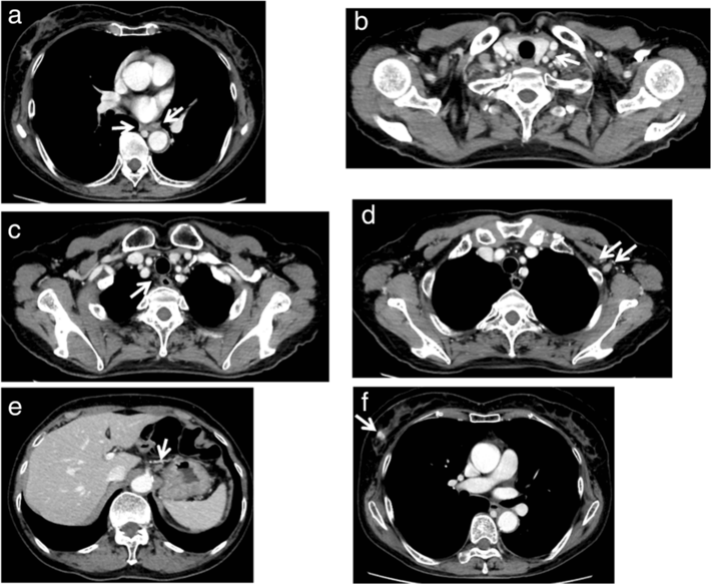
Chest and abdominal computed tomography after four courses of chemotherapy with docetaxel, cisplatin, and 5-fluorouracil. a The wall thickening in the middle thoracic esophagus had almost disappeared. b The size of the left supraclavicular lymph node did not change (8.0 mm in the short-axis plane). c The size of the right recurrent nerve lymph node had reduced (3.3 mm in the short-axis plane). d The size of the lymph node metastases in the left axillary space had reduced (9.3 and 2.1 mm in the short-axis plane). e The size of the lymph node along the lesser curvature of the stomach had reduced (5.7 mm in the short-axis plane). f The mass of the right breast had reduced in size (maximum diameter of 13.6 mm)
